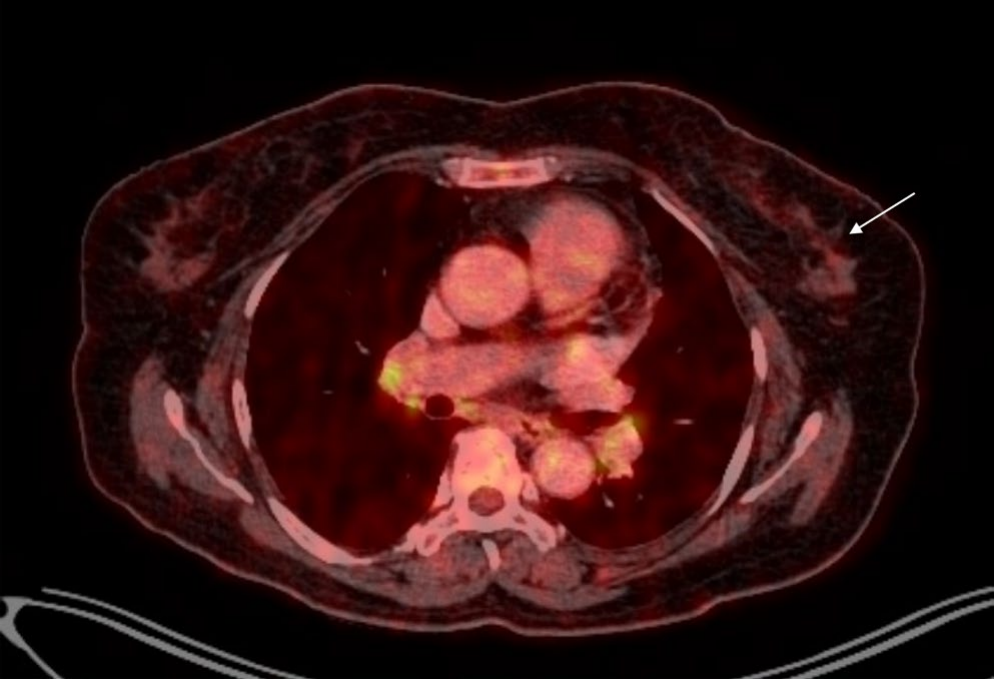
Response assessment positron emission tomography/computed tomography (PET/CT) post 6 months of treatment showing non‐FDG avid heterogeneously enhancing soft tissue density lesion in the left breast with resolution of metabolic activity (white arrow).
